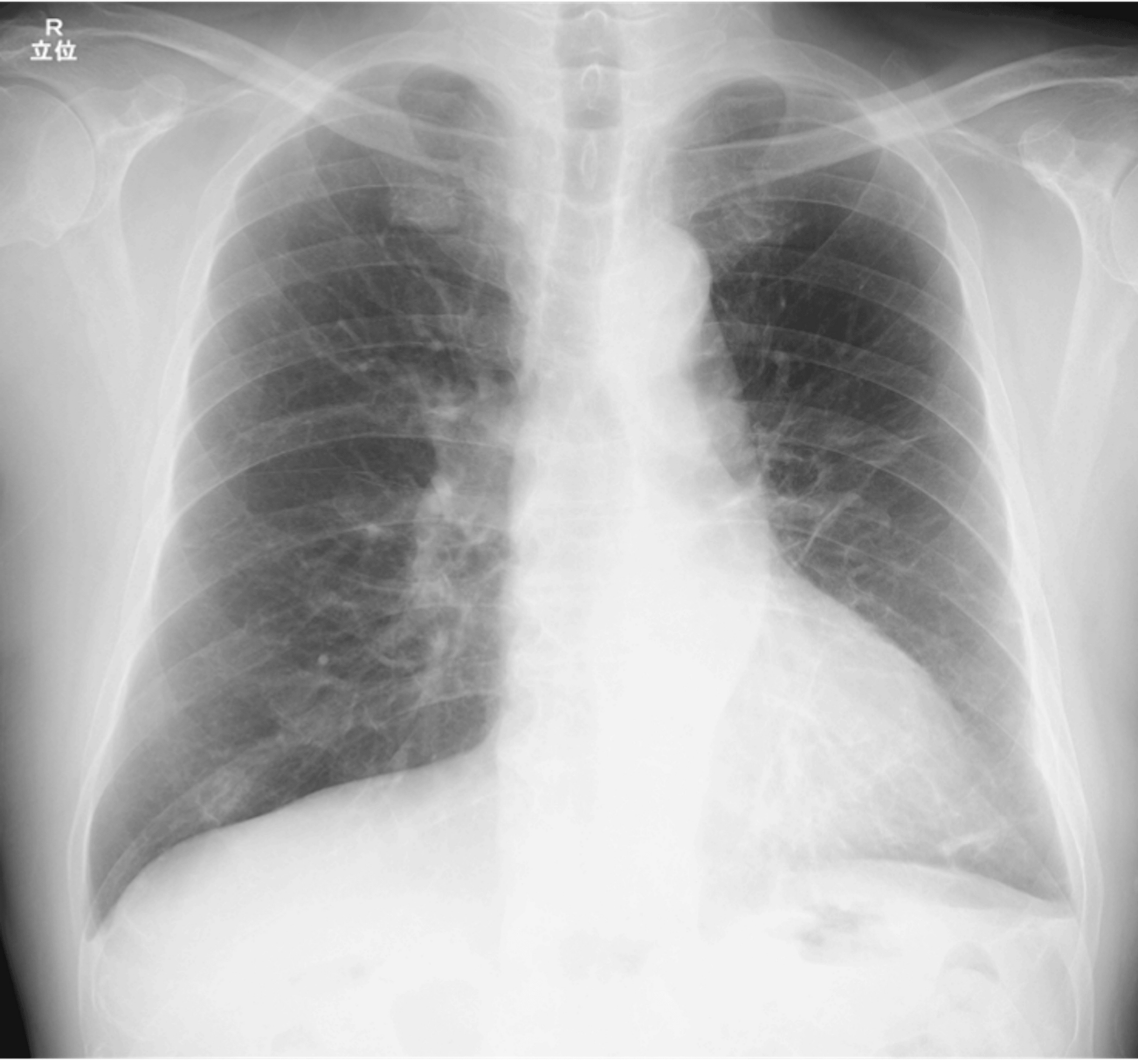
Chest radiograph on Day X+65 revealing near-complete resolution of the abnormal shadows.
